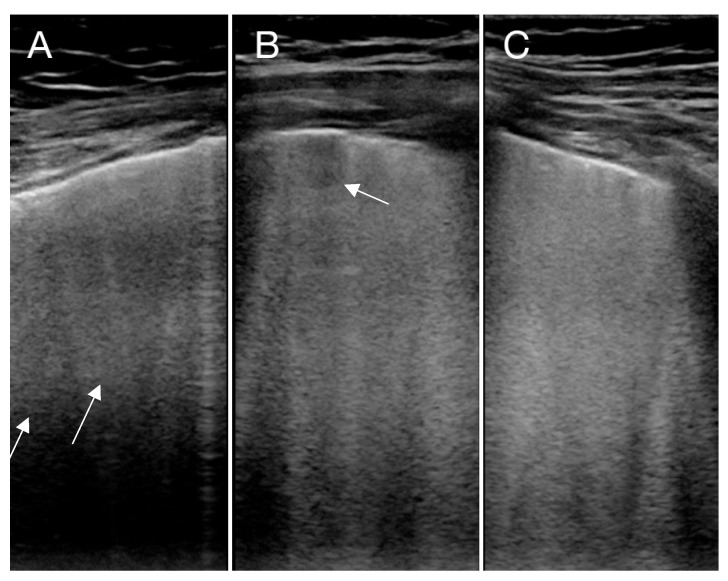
Lung ultrasound (LUS) of three different patients with COVID-19 showing multifocal (A,B) (marked with an arrow) and confluent B-lines (C). In (B), a small subpleural consolidation is also seen (marked with an arrow).
